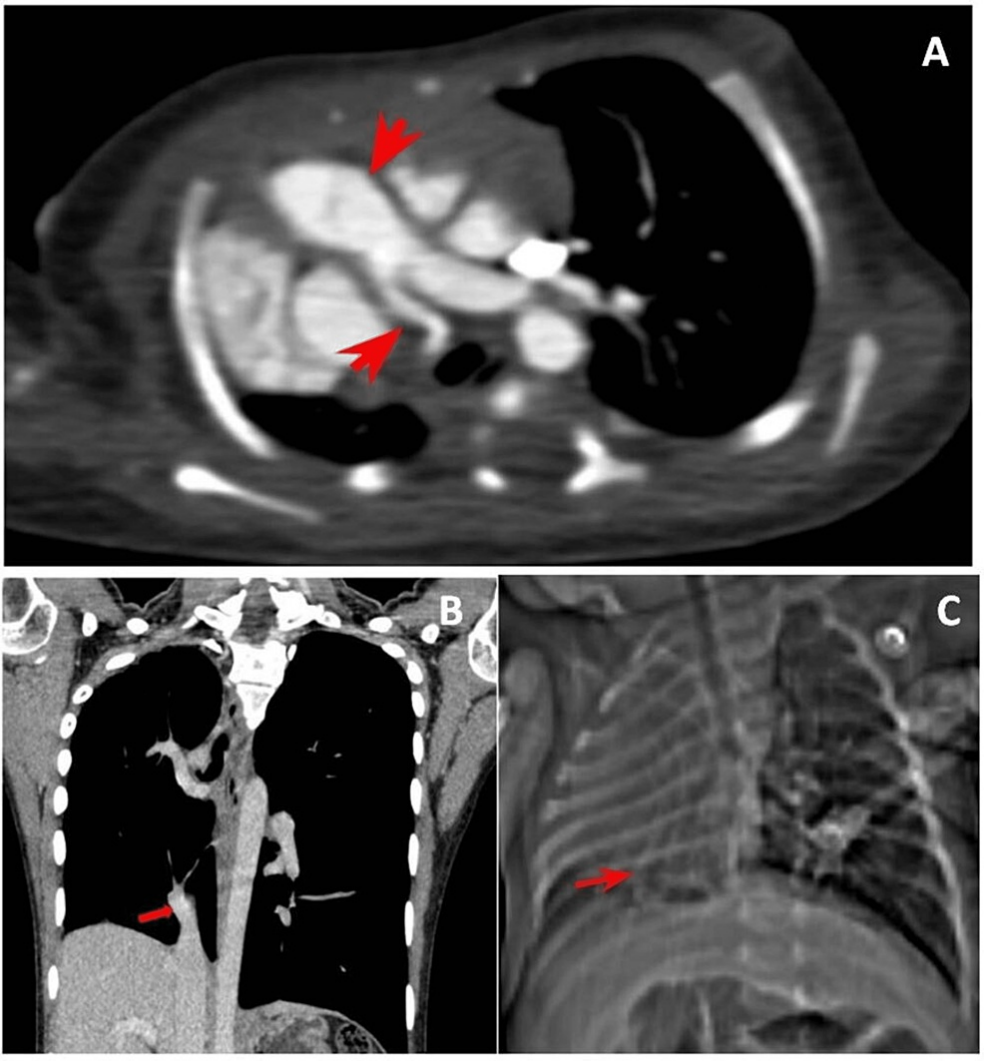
Hypoplastic right pulmonary artery with PAPVR forming the scimitar sign A 15-year-old female patient presented with complaints of repeated URTI. On further evaluation, hypoplastic right lung field was noted on Chest Radiograph. On Chest CECT evaluation, hypoplastic right pulmonary artery was noted with an anomalous vein draining into the IVC forming the scimitar sign. 4A: Chest CECT of the patient demonstrating a hypoplastic right pulmonary artery (red arrows). 4B: Chest CECT of the patient demonstrating an anomalous vein draining into the IVC (red arrow) suggestive of PAPVR - scimitar sign. 4C: Chest X-Ray of a 20-day neonate showing the scimitar sign (red arrow). PAPVR: Partial Anomalous Pulmonary Venous Return, URTI: Recurrent Upper Respiratory Tract Infection, IVC: Inferior Vena Cava
